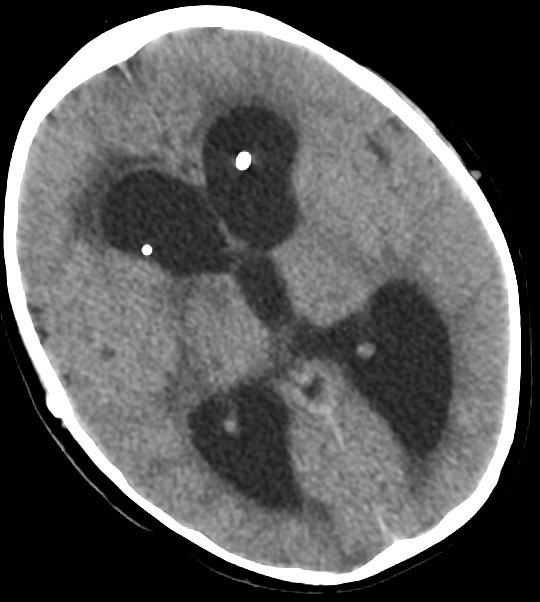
Preoperative CT scan demonstrating hydrocephalus
